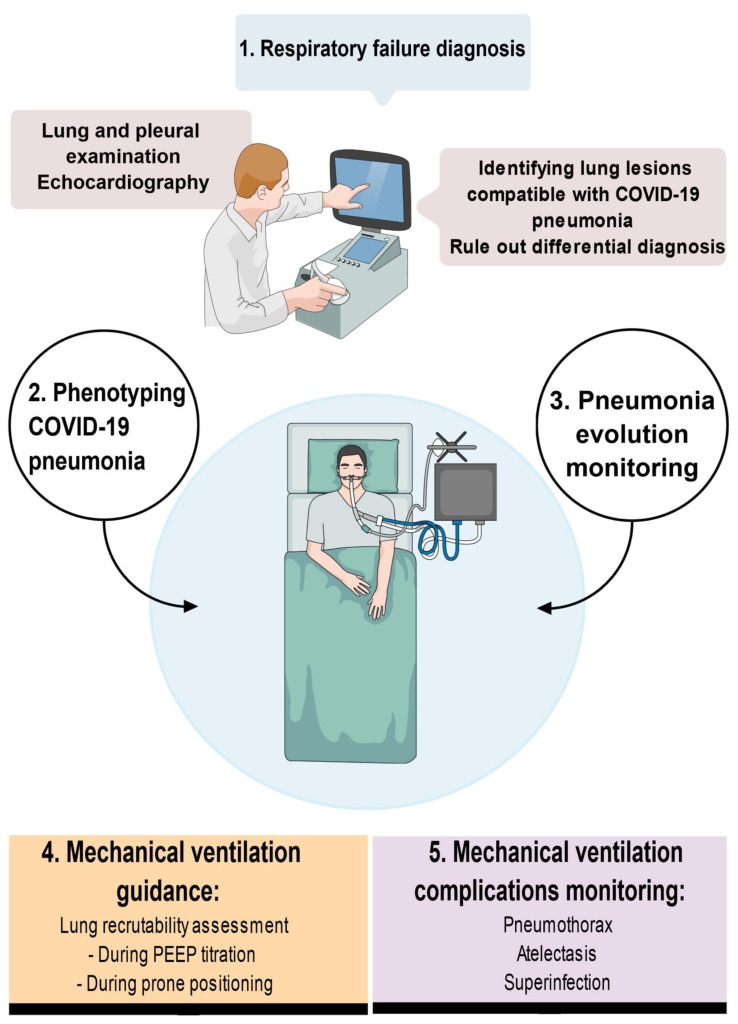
Scope of lung ultrasound in patients admitted with acute respiratory failure and suspected COVID-19 pneumonia.
